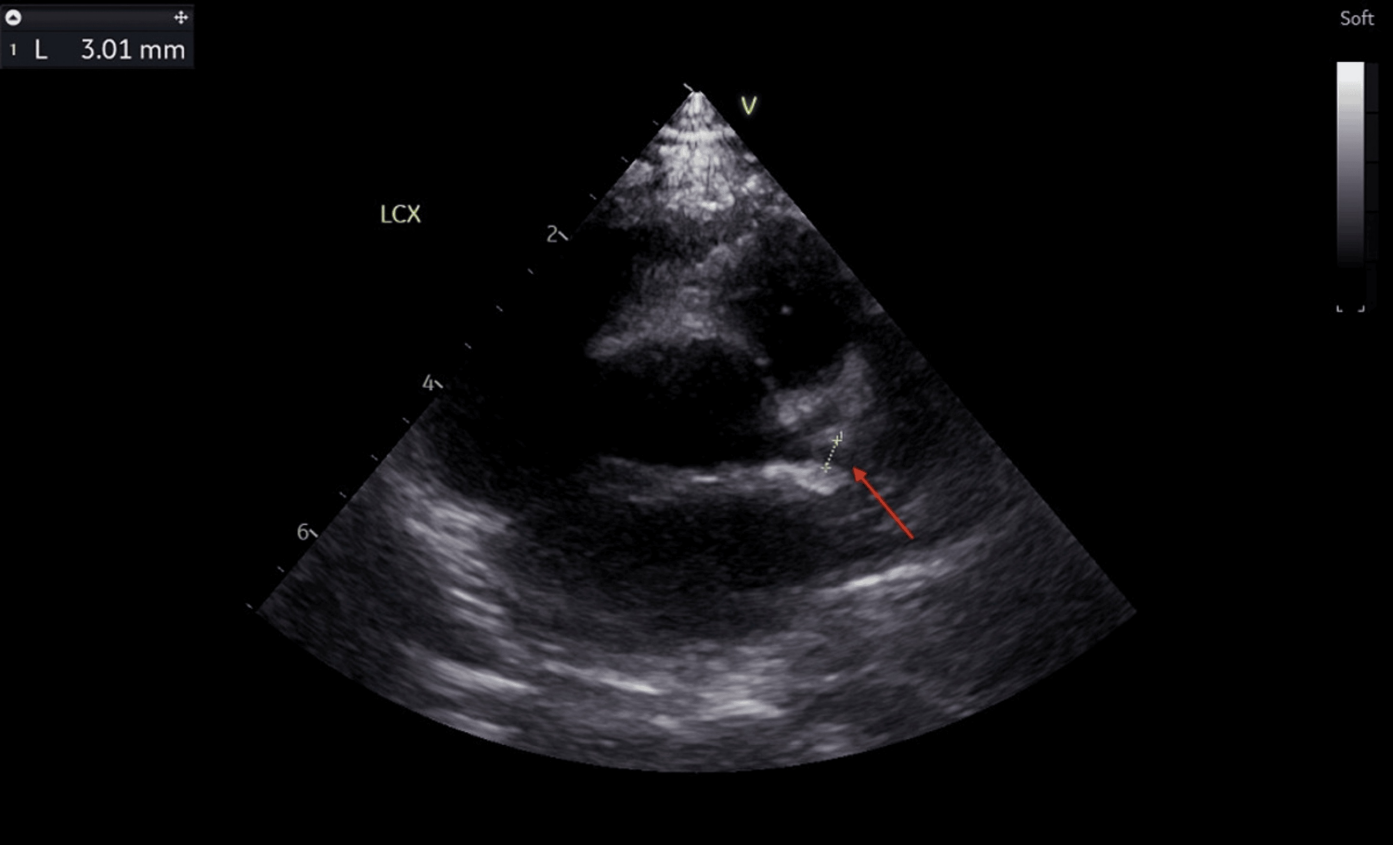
Echocardiogram showing a dilated left circumflex artery. The red arrow points at the dilated left circumflex artery measuring 3.0 mm (Z score: +5.11).
